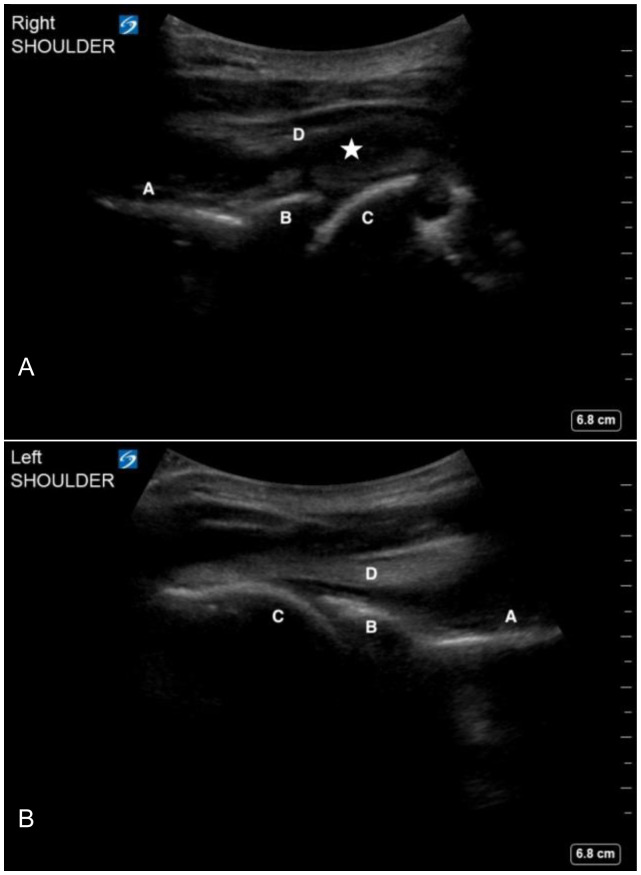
Ultrasound images of the affected right shoulder (2A) and unaffected left shoulder (2B) showing scapular spine (A), glenoid (B), humeral head (C) and infraspinatus tendon (D). A mixed-echotexture collection consistent with an intraarticular hemarthrosis (star) is visualized in the right shoulder.
